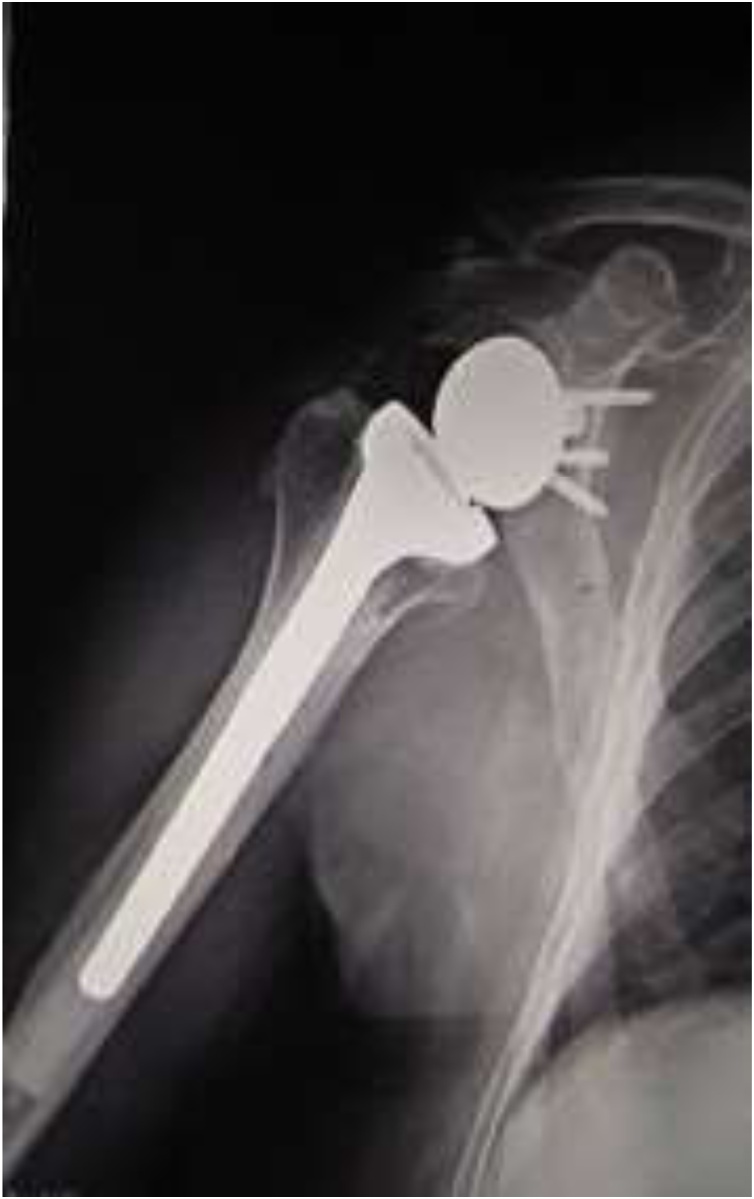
Postoperative radiographs of the RTSA.
